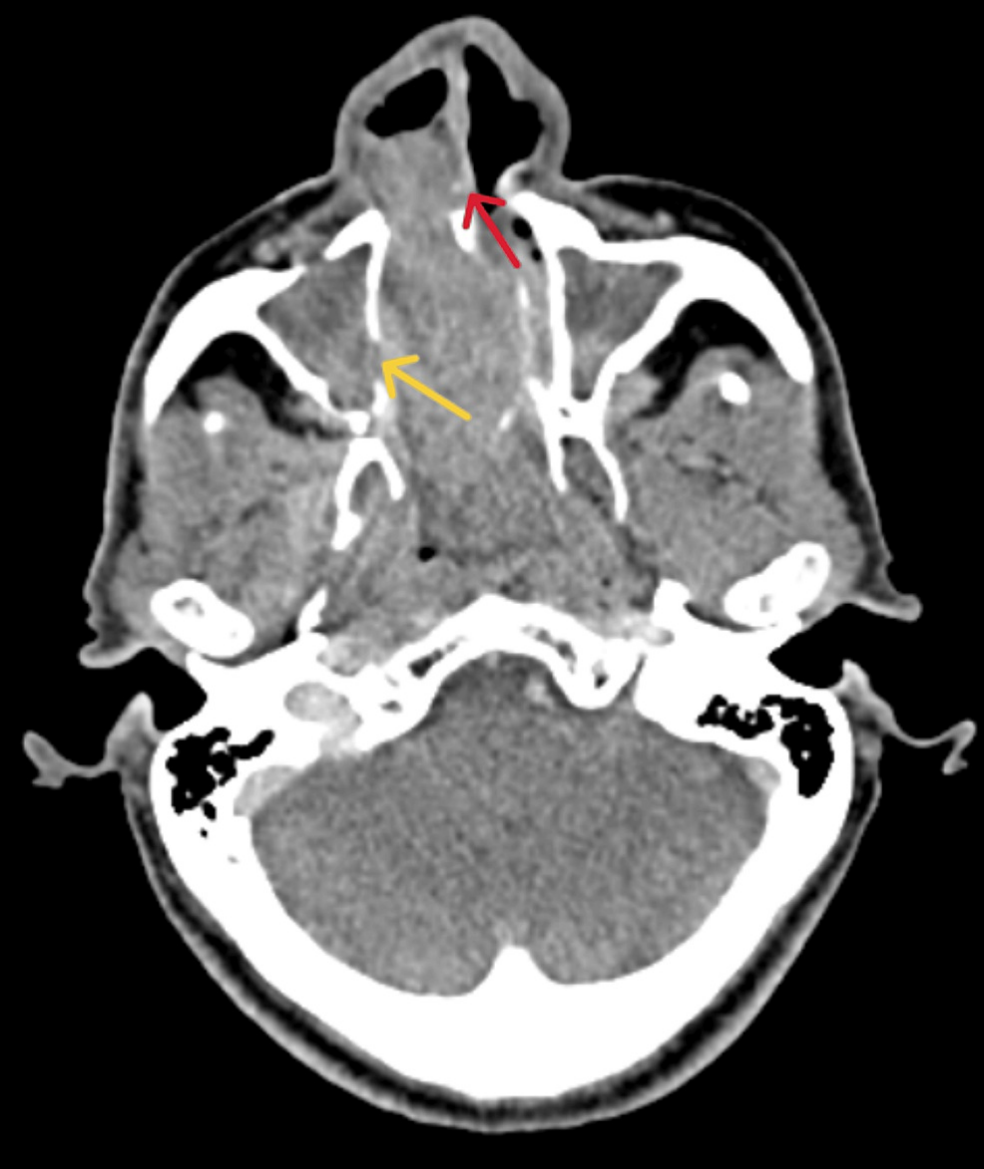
CT paranasal sinus (axial view) CT- Computed tomography A large soft tissue density mass lesion is seen in both nasal cavities, mainly in the right nasal cavity, resulting in near complete opacification with destruction of the nasal septum (red arrow) and widening of both maxillary ostia with destruction of right side maxillary wall (yellow arrow) and lamina papyracea. Complete opacification of all sinuses except left frontal sinus findings are suggestive of sinonasal mass as inverted papilloma.
